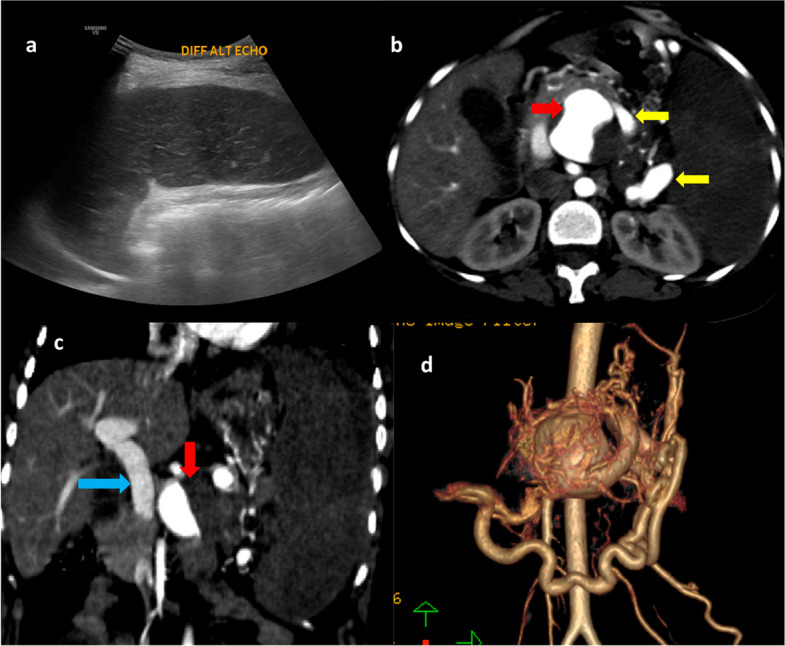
A Ultrasound of the abdomen shows a markedly hypoechoic spleen. B, C Axial and coronal CECT images demonstrate a partially thrombosed splenic artery aneurysm (red arrow) with early arterial opacification of the splenic vein (yellow arrows in B) and portal vein (blue arrow in C), consistent with a splenic arteriovenous fistula. D 3D volume-rendered reconstruction depicts the splenic artery aneurysm with markedly dilated splenic (arrow) and gastric veins
